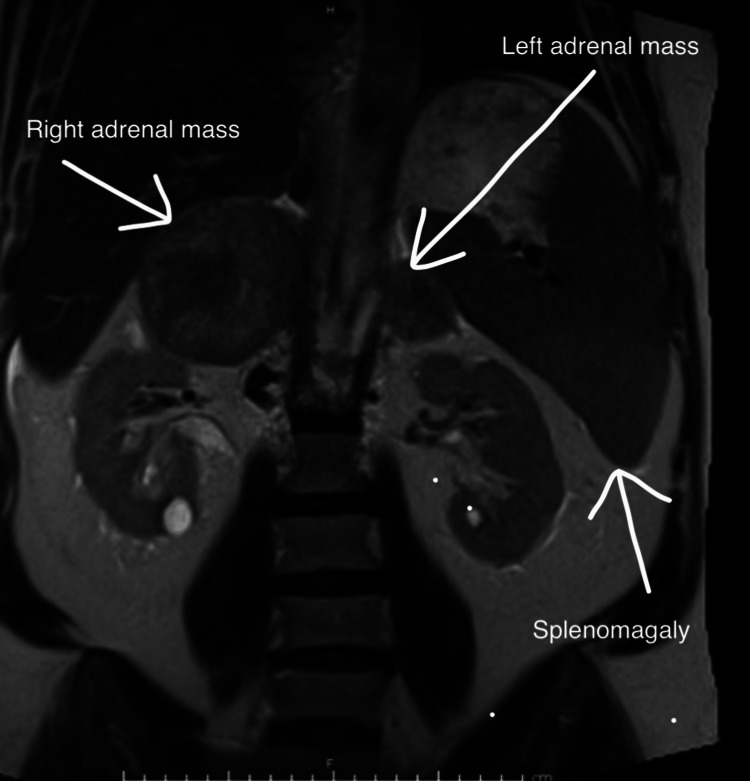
T2-weighted (T2W) MRI abdomen and pelvis Confirmed bilateral adrenal masses, with the right adrenal mass now measuring 8.5 cm. Splenomegaly was present. Features were suggestive of lymphoma versus metastases from an unknown primary. Multidisciplinary team (MDT) discussion was advised, and a biopsy was arranged and performed.
